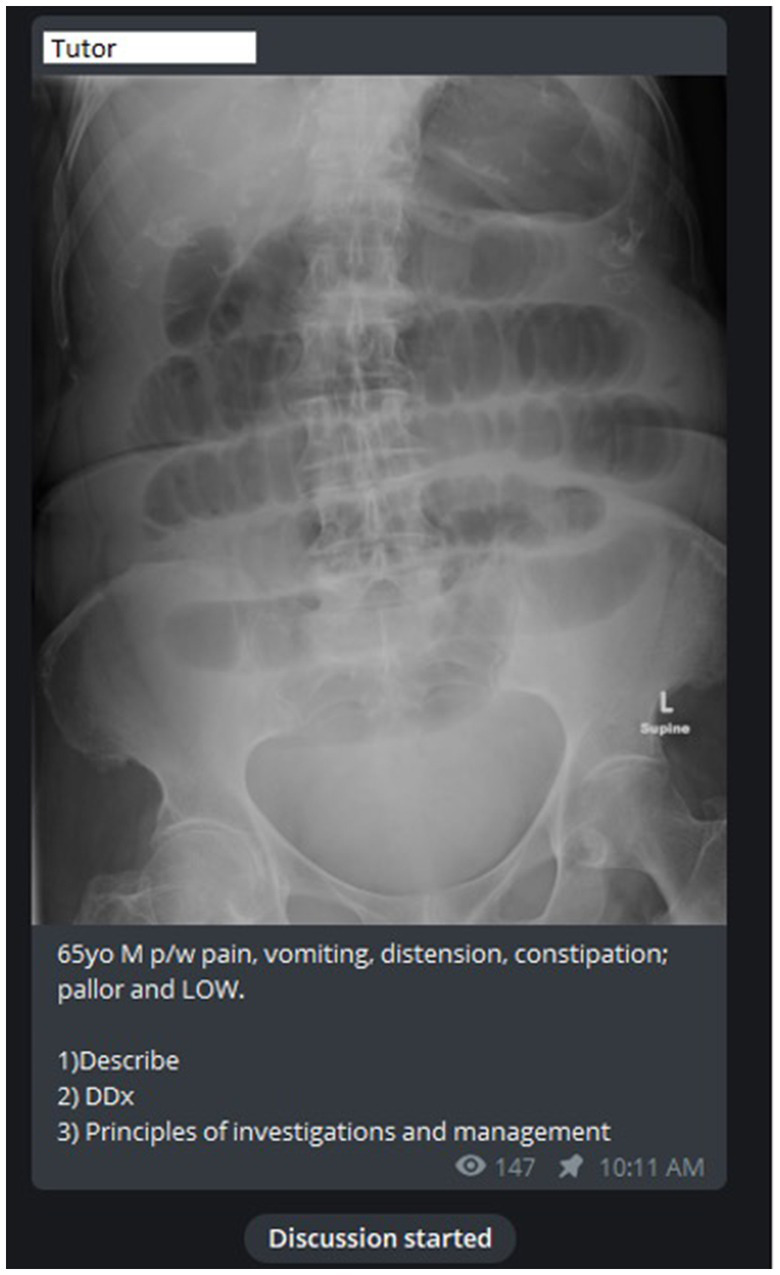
Case discussion with an abdominal radiograph of a 65 year old male. P/W: Presenting with; DDx: Differential diagnoses.
